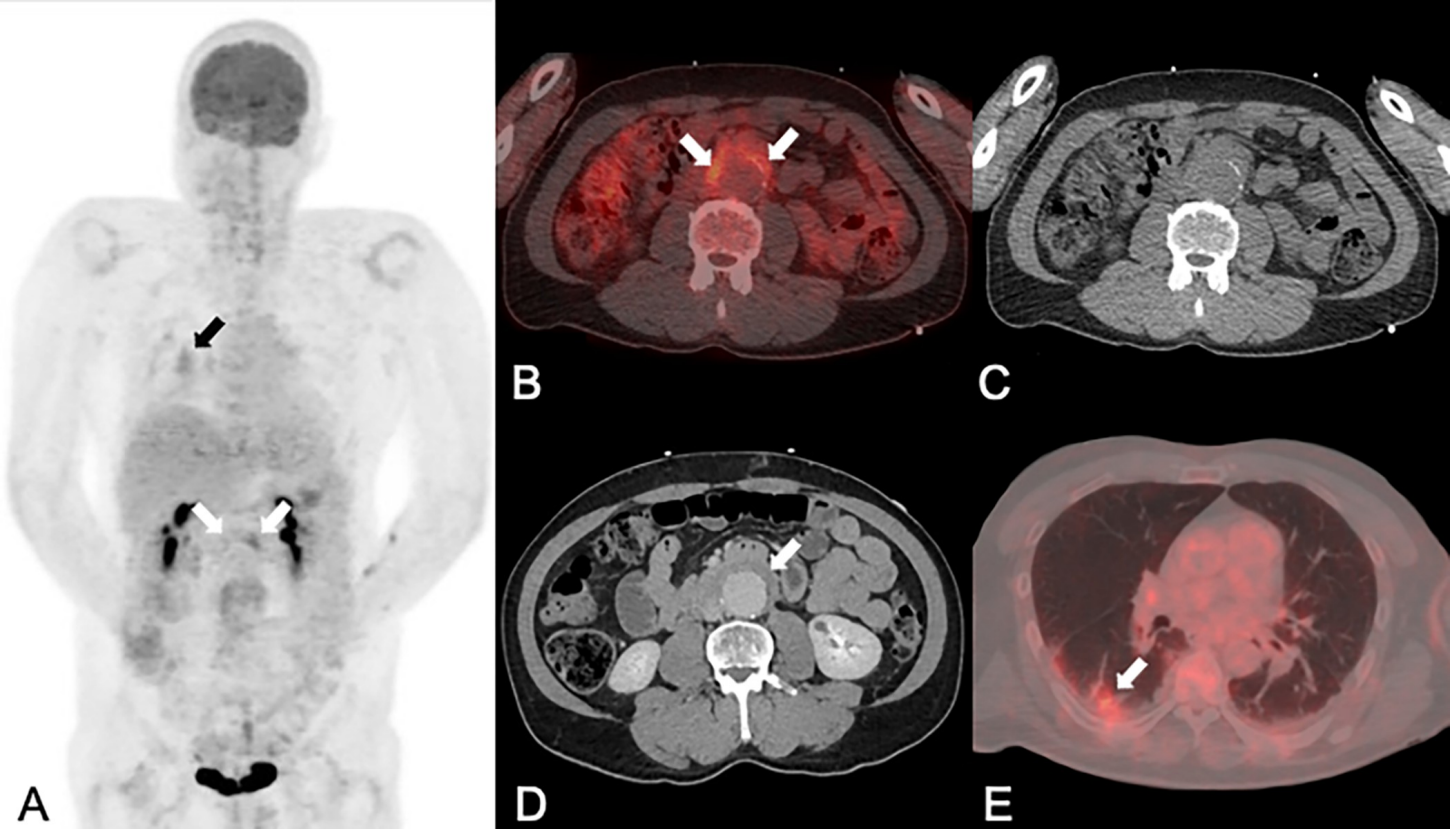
A 51-year-old man with an inflammatory abdominal aortic aneurysm, initially presenting with back pain and abdominal pain. PET/CT (A: maximum intensity projection of PET; B and E: fused PET/CT images) and contrast-enhanced CT (CE-CT: D) showed a 52 mm large abdominal aortic aneurysm (white arrows in B and D) with increased FDG uptake (SUVmax 3.6 in A and B) in the thickened (12 mm) aortic wall (white arrow in D) with dorsal sparing. Using PET/CT images alone (A-C) one reader correctly diagnosed an inflammatory aneurysm, while the other one falsely diagnosed an infectious/mycotic aneurysm (notably, wall thickening may not be clearly delineated on the non-enhanced CT part of the PET/CT in C, but only on the CE-CT in D). With CE-CT (E) and with combined imaging (not shown) both readers correctly diagnosed an inflammatory aneurysm. As an incidental finding, a small metabolically active pneumonia was diagnosed on PET/CT images (E). The patient was subsequently treated with percutaneous endovascular abdominal aortic aneurysm repair and steroid therapy. The latest follow-up imaging five years after the initial diagnosis (not shown), showed a stable abdominal aortic graft with no relevant wall thickening, and the patient had no abdominal symptoms.
